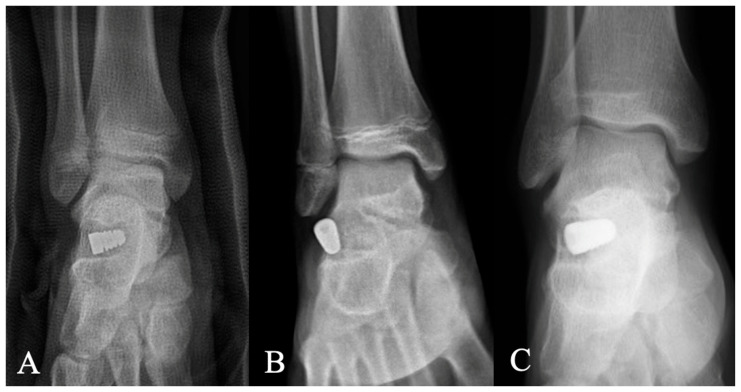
Early implant dislodgement in a 13-year-old boy who sustained an ankle sprain 1 month postoperatively. (A) An immediate postoperative AP radiograph demonstrating appropriate implant placement. (B) A follow-up radiograph at 1 month showing dislodgement of the implant with loss of correction. (C) Five-year radiographic follow-ups showed the implant in a good position.
